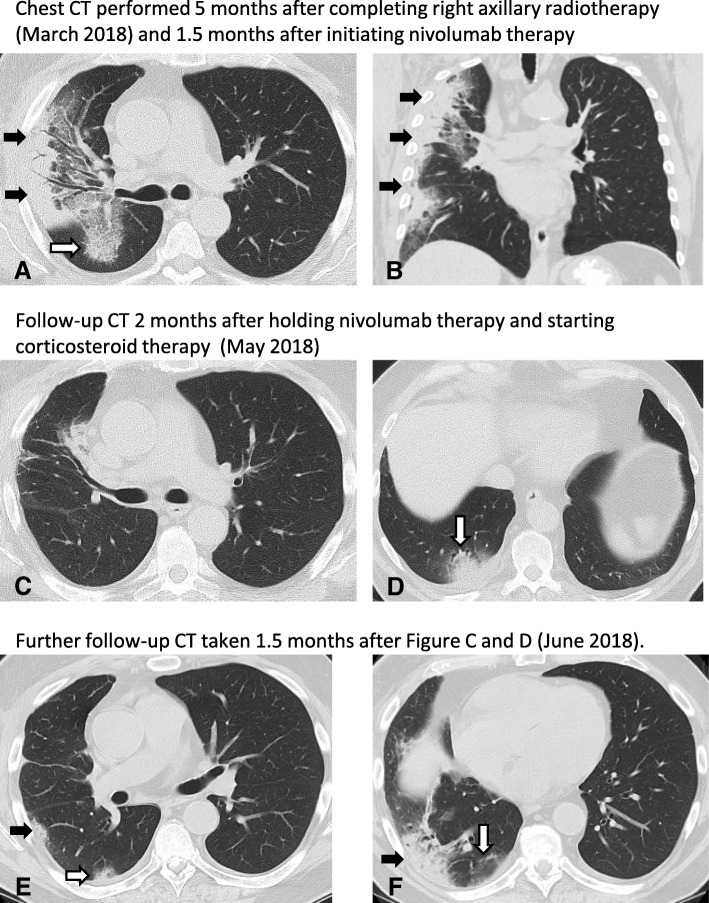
a, b. CT scan of the chest demonstrated peripheral curvilinear consolidative opacities predominantly in the right upper lobe (black arrows, a, b), accompanied by more central areas of ground glass opacities (GGO) and traction bronchiectasis. Most of the findings are within the radiation field, however, a focal area of GGO extended posteriorly into the superior segment of the right lower lobe (white arrow, a) which is outside of the radiation field. c, d. On a follow-up CT scan of the chest performed 2 months after A and B, previously noted peripheral consolidative opacities and GGO were mostly resolved, in response to corticosteroid therapy (c). However, a new focus of peripheral consolidation with surrounding GGO was noted in the right lower lobe (white arrow, d) outside of the irradiated lung field. e, f. Further follow-up CT taken 1.5 months after Fig. c and d demonstrated resolving peripheral consolidation that appeared on Fig. D noted as residual GGO (white arrow, f); however, additional new foci of peripheral consolidation with surrounding GGO are noted both outside of the radiation field (white arrows, e) and within the irradiated lung (black arrows, e, f)
